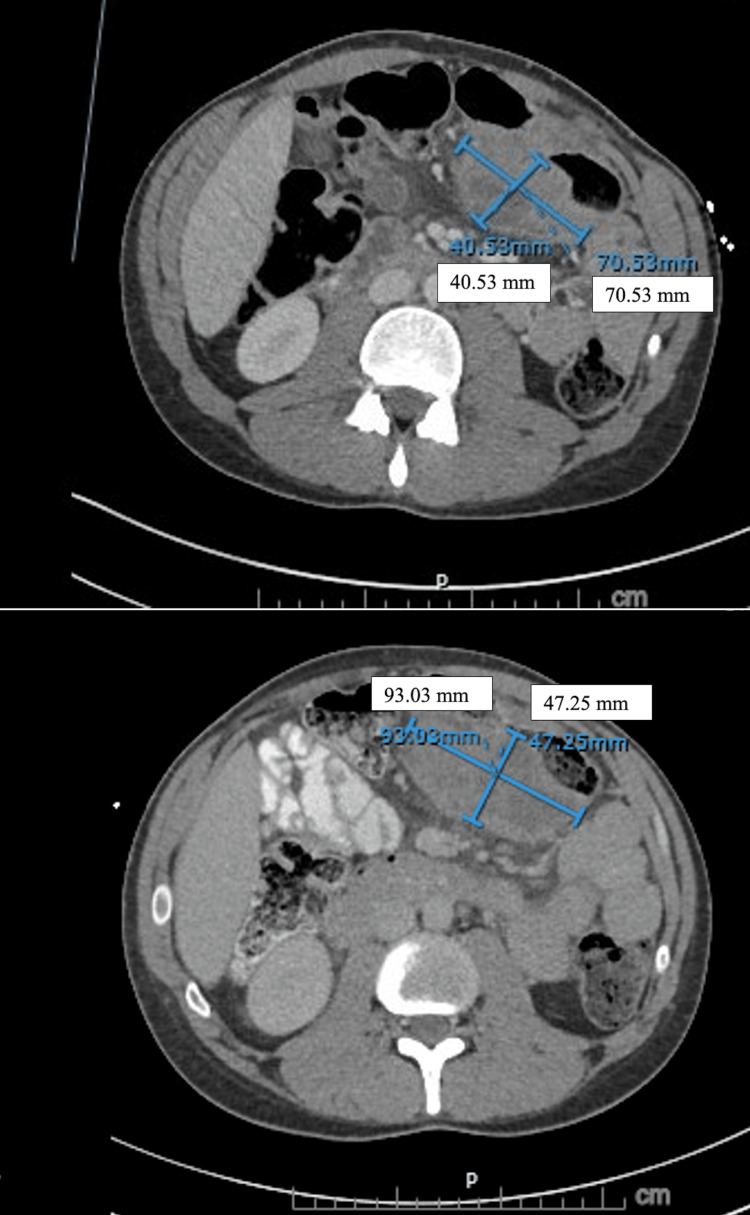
This CT abdomen/pelvis shows rapid disease progression Seen is a mass on the left upper quadrant encasing the segment of the transverse colon. The image on top is of the initial CT, where the mass measures 70.53 mm x 40.53 mm. The image at the bottom is from the follow-up CT performed 20 days after the initial CT, revealing an increase in the mass size (93.03 mm x 47.25 mm).
